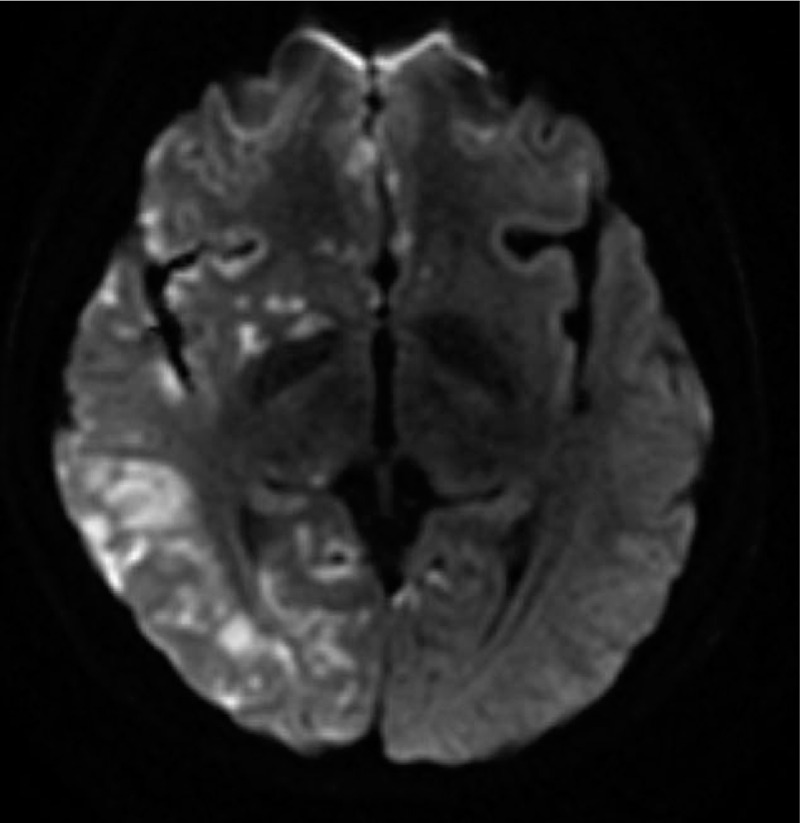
Brain MRI showing paradoxical CO_2_ embolism. The diffusion-weighted imaging (DWI) image revealed multiple cerebral infarction, predominantly on the right side of the cerebral cortex on day 5 after the procedure. MRI = magnetic resonance imaging.
